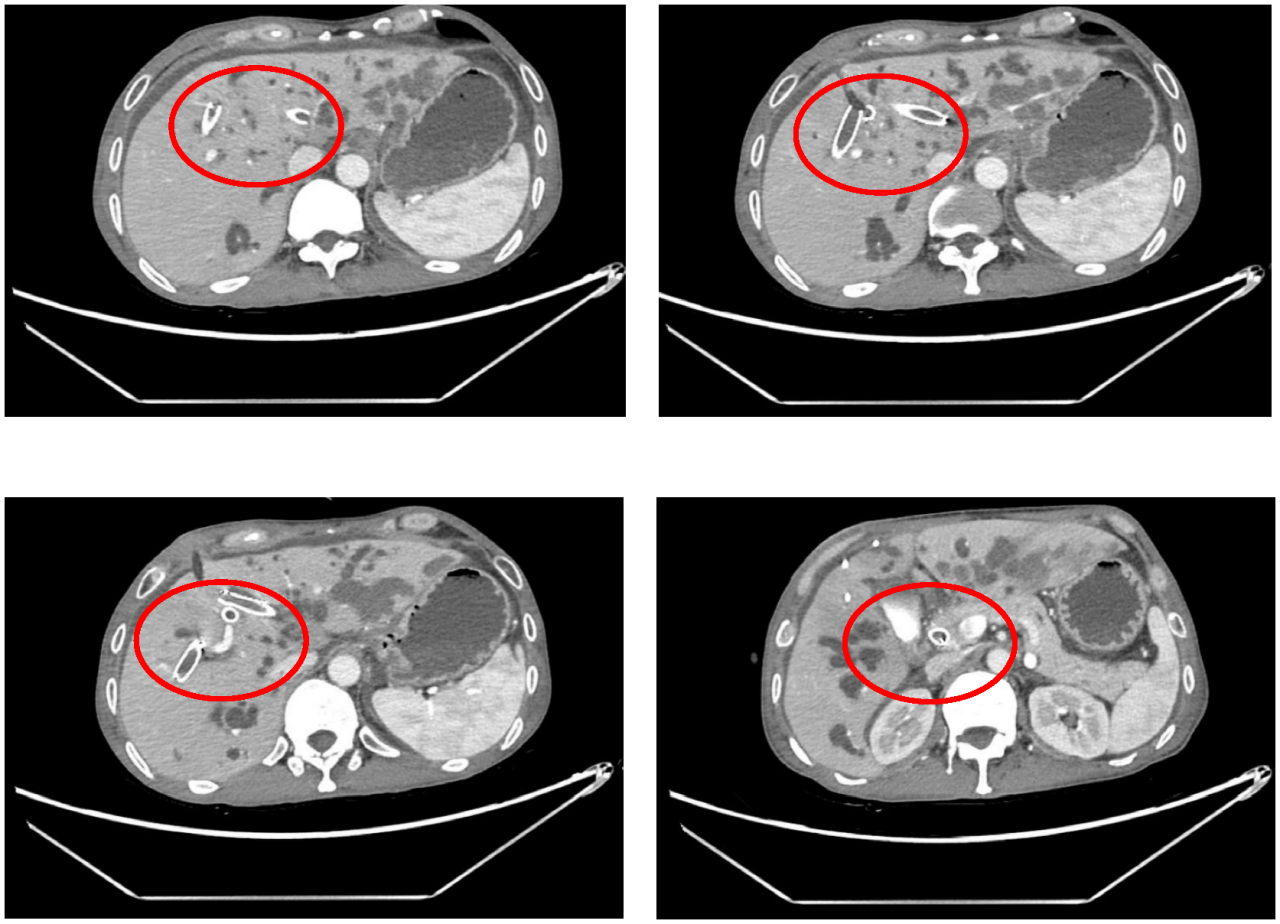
Computed tomography showed no migration of the stents.
